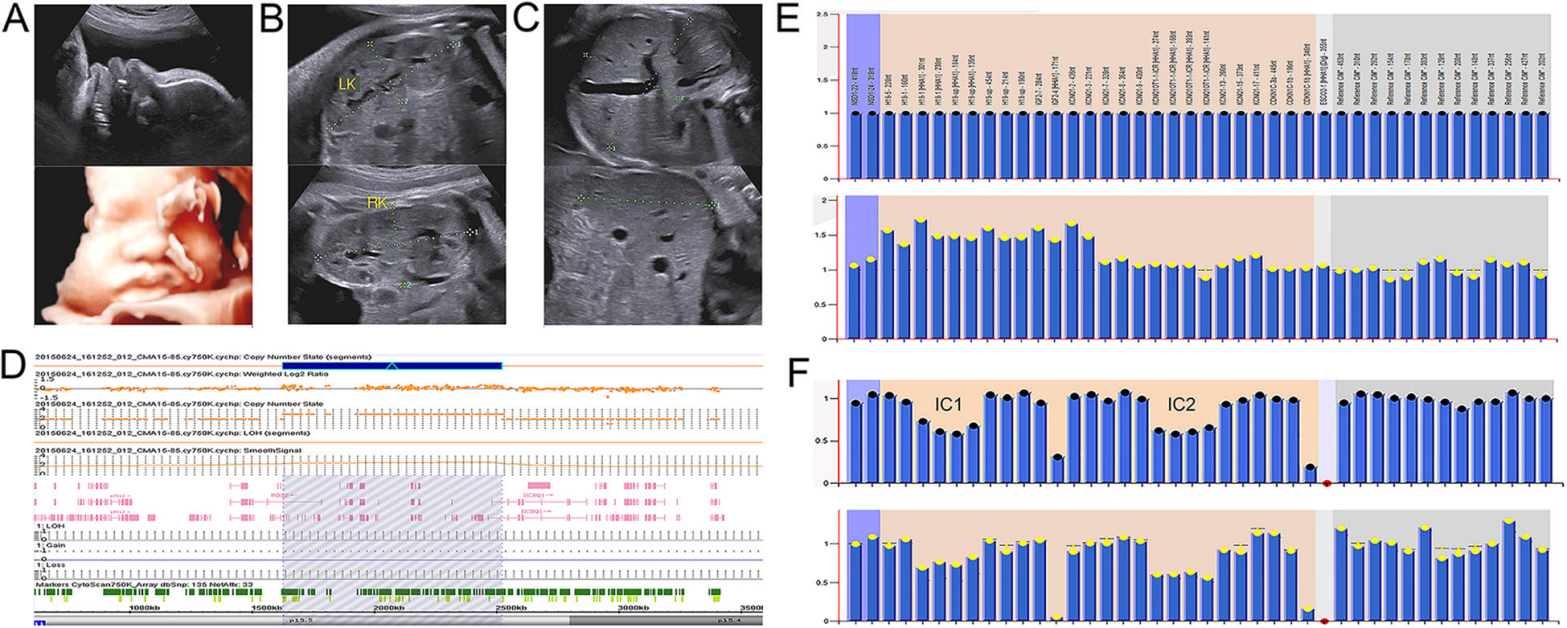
Prenatal ultrasound images at 34 weeks of gestation, CMA and MS-MLPA results in patient 1. a 2D and 3D prenatal ultrasound examinations at 34 weeks demonstrated macroglossia. b Nephromegaly at 34 weeks prenatal ultrasound image with left kidney length of 7.89 × 3.39 × 3.67 cm, right kidney length of 6.86 × 3.59 × 3.55 cm. c Hepatomegaly at 34 weeks prenatal ultrasound image with length of 5.46 × 8.18 × 5.28 cm. d The CMA chromosome view (up) and gene view (bottom) reveal the breakpoint location and an 896Kb duplication at 11p15.5 (arr[GRCh37]11p15.5(1,632,167–2,527,910)×3). e MS-MLPA shows a peak height ratio value of 1.5 (three copies) at 11p15 (bottom) in comparison with a ratio value of 1 (two copies) from a normal control (upper). f MS-MLPA indicates methylation index of 0.76 at IC1 and methylation index of 0.61 at IC2 (bottom) in comparison with normal control methylation index of 0.65 at IC1 and 0.62 at IC2 (upper)
